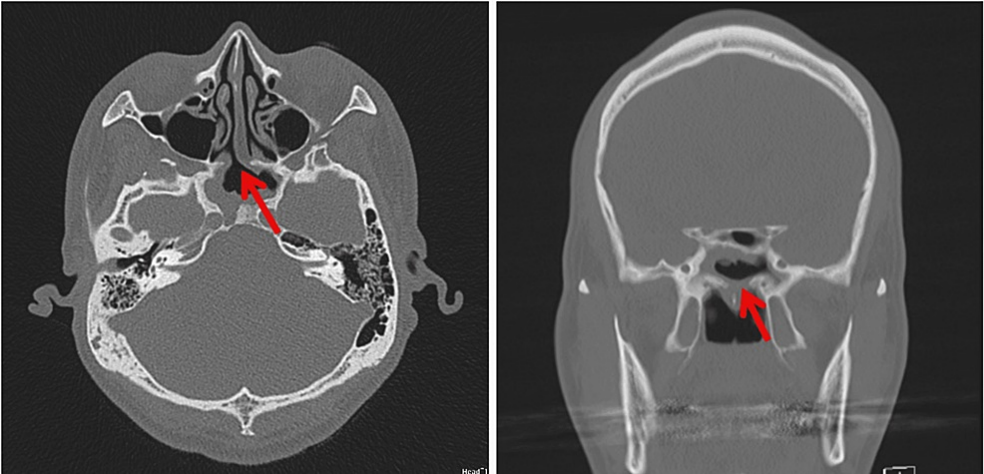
Radiographic examination of the skull base before orthognathic surgery. Left panel: sagittal CT scan demonstrating a bony defect in the sella turcica (red arrow). Right panel: coronal CT scan demonstrating the same bony defect (red arrow).
